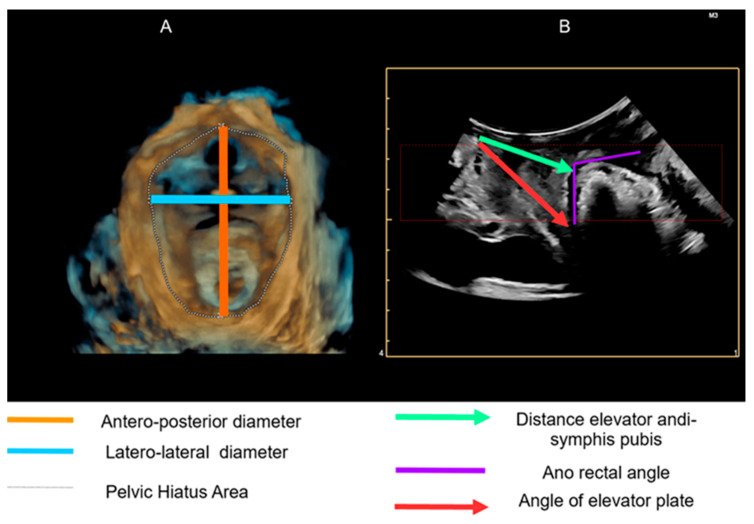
Evaluation of the pelvic floor hypertonus through objective measurement of selected parameters using trans-labial ultrasound with a 4D Convex Probe. (A): 3D reproduction; (B): 2D reproduction.
